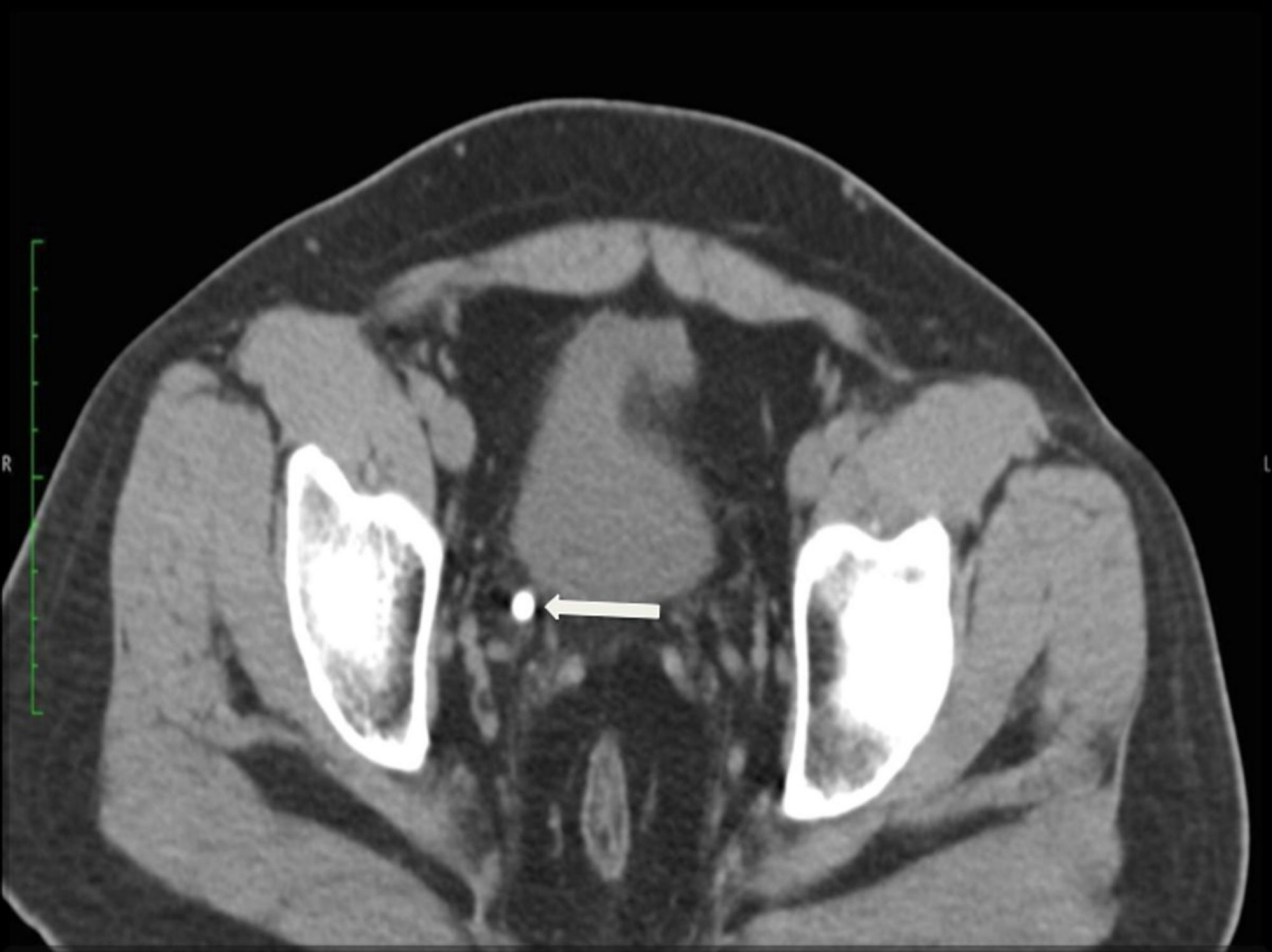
CT KUB (axial view) - Revealed bladder calculi (arrow). CT: Computed tomography; KUB: Kidney ureter bladder.
